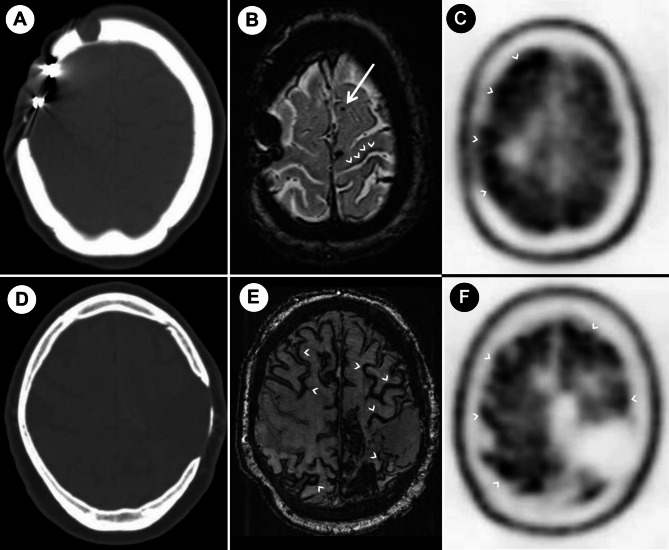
Patient 1: computed tomography showing right-sided fronto-parietal cranial bone defect related to neurosurgical treatment (A); susceptibility weighted magnetic resonance imaging showing cortical microbleeds (arrow) and cortical superficial siderosis (arrowheads) (B); amyloid 18 F-flutemetamol-PET showing a diffuse right-side predominant (arrowheads) supratentorial cortical uptake (C). Patient 2: computed tomography showing left-sided fronto-parietal cranial bone defect related to neurosurgical treatment (D); susceptibility weighted magnetic resonance imaging showing severe cortical superficial siderosis (arrowheads) (E); amyloid 18 F-flutemetamol-PET showing diffuse supratentorial cortical uptake (arrowheads) (F)
